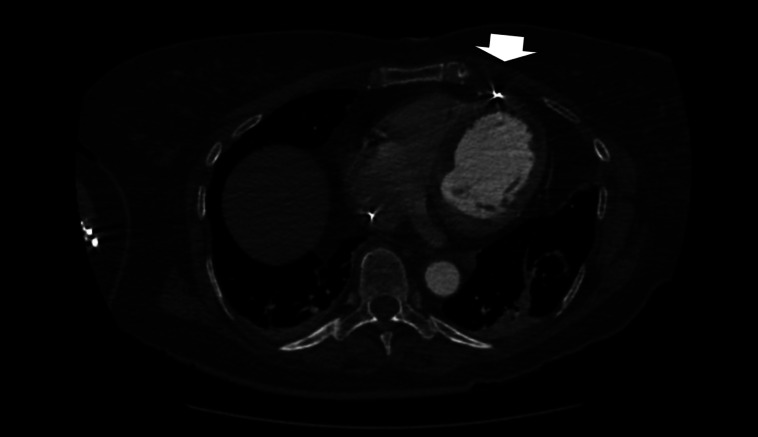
Computed tomography chest showing the right ventricular lead tip perforating into the pericardiac fat (arrow).
